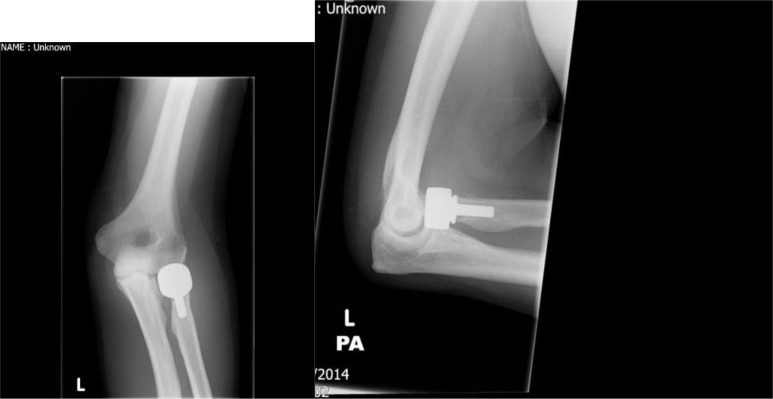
Post-operative plain radiographs after arthroplasty of type III fracture.
